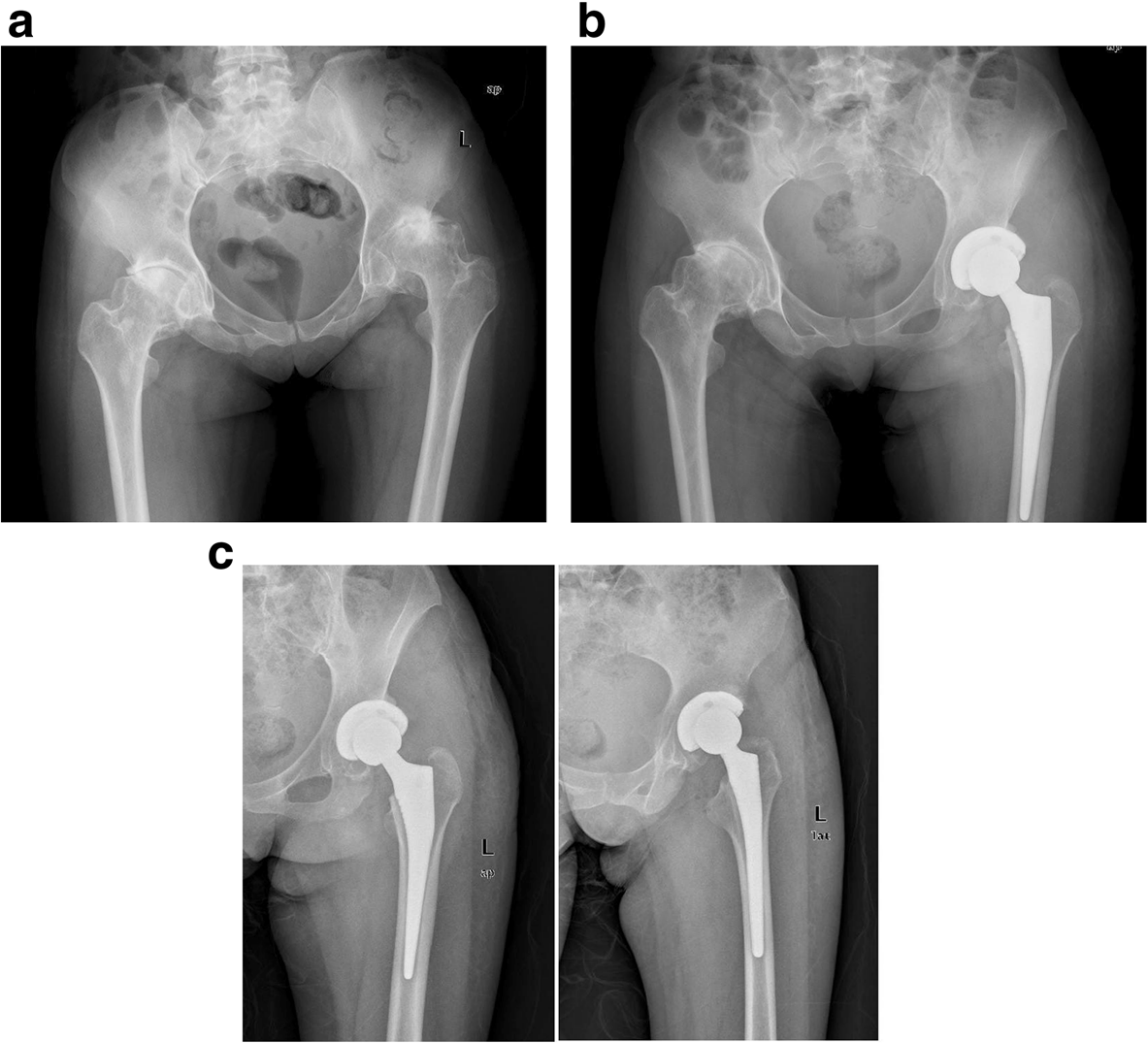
A male patient (bilateral hip with KBD) (a) with scores of 70, 43 and 25 points in the left hip on the VAS, Harris and Fast-KBD scales, respectively. He received total hip replacement in his left hip (b). Five-and-a-half years after the operation, there was no radiographic evidence of loosening or changes in component positioning (c), with scores of 10, 91 and 36 on the VAS, Harris and FSAT-KBD scales, respectively
